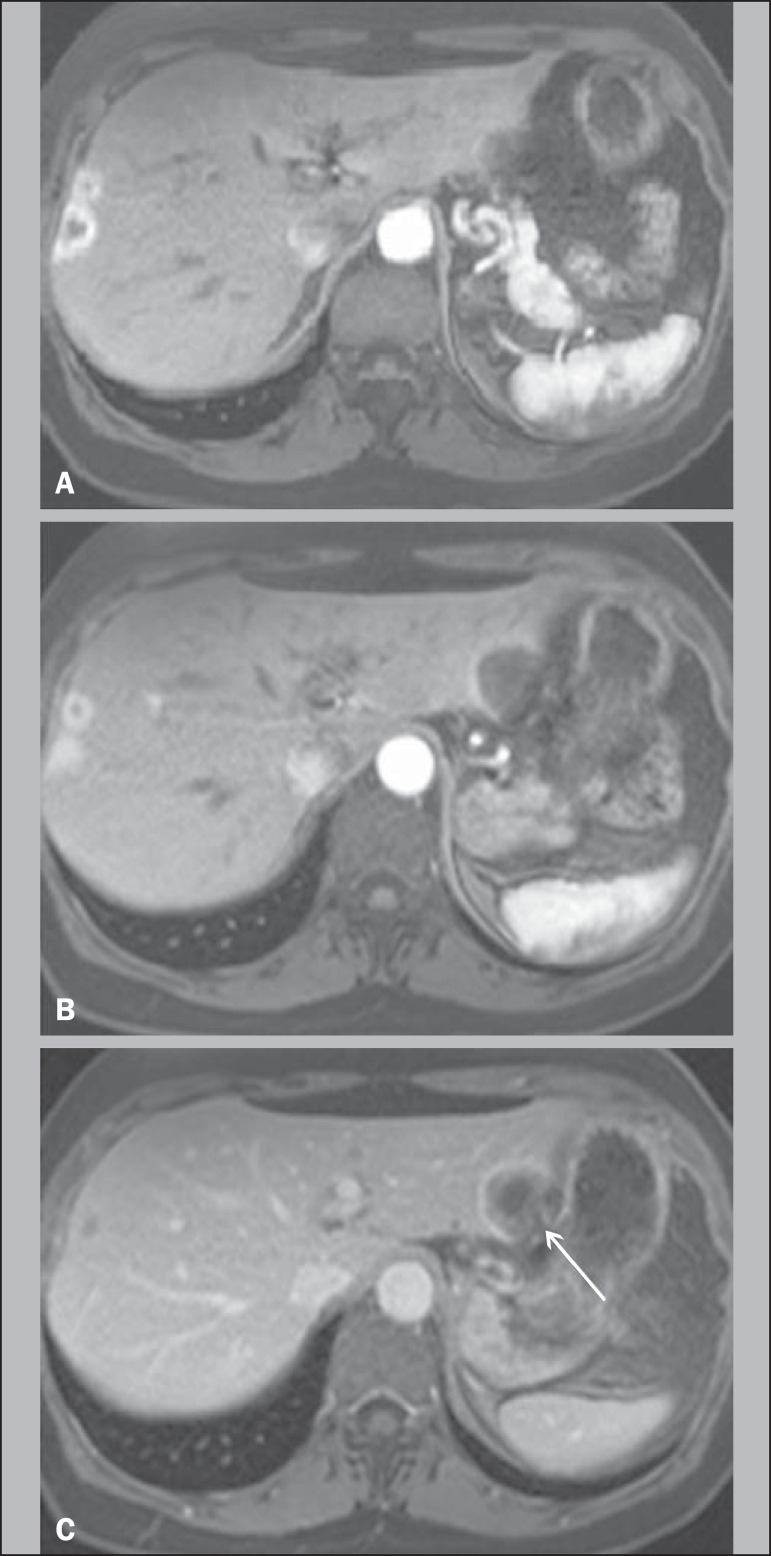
A 64-year-old female with HEHE. Axial, postcontrast T1-weighted
